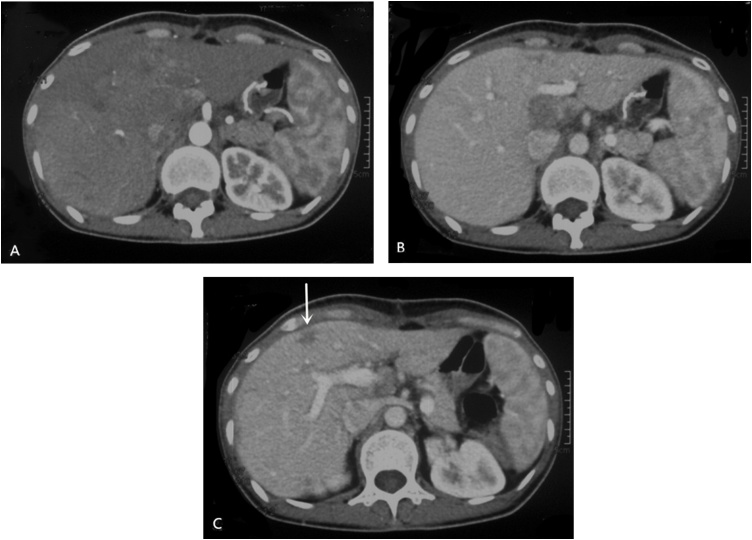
Abdominal computed tomography revealed that the tumor with stable disease after two courses of FOLFOX chemotherapy via the portal vein in October 2011.
